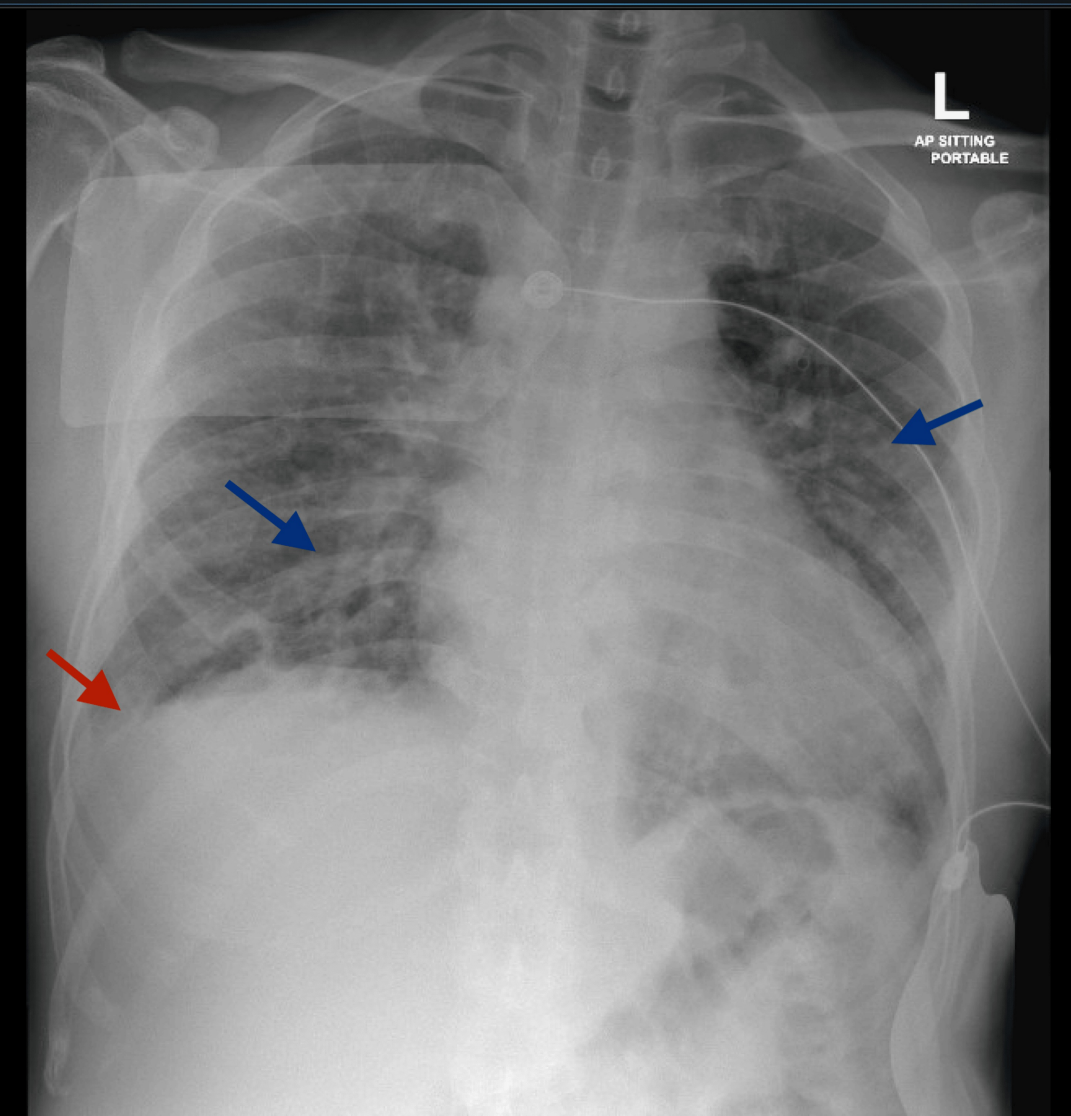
CXR showing increased broncho-vascular markings (blue arrows) and right sided pleural effusion (red arrow) CXR - Chest X-Ray
